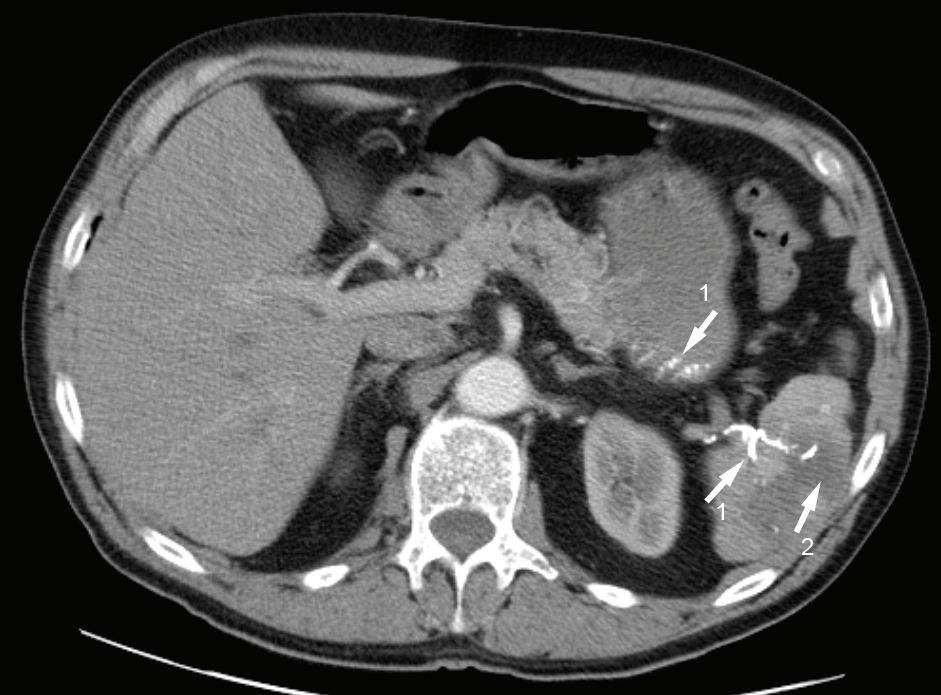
Axial contrast enhanced CT scan of the upper abdomen showing lipiodol in the arterial collaterals of the stomach running to the spleen (1). Furthermore, CT revealed an infarction of splenic tissue adjacent to these vessels (2).
